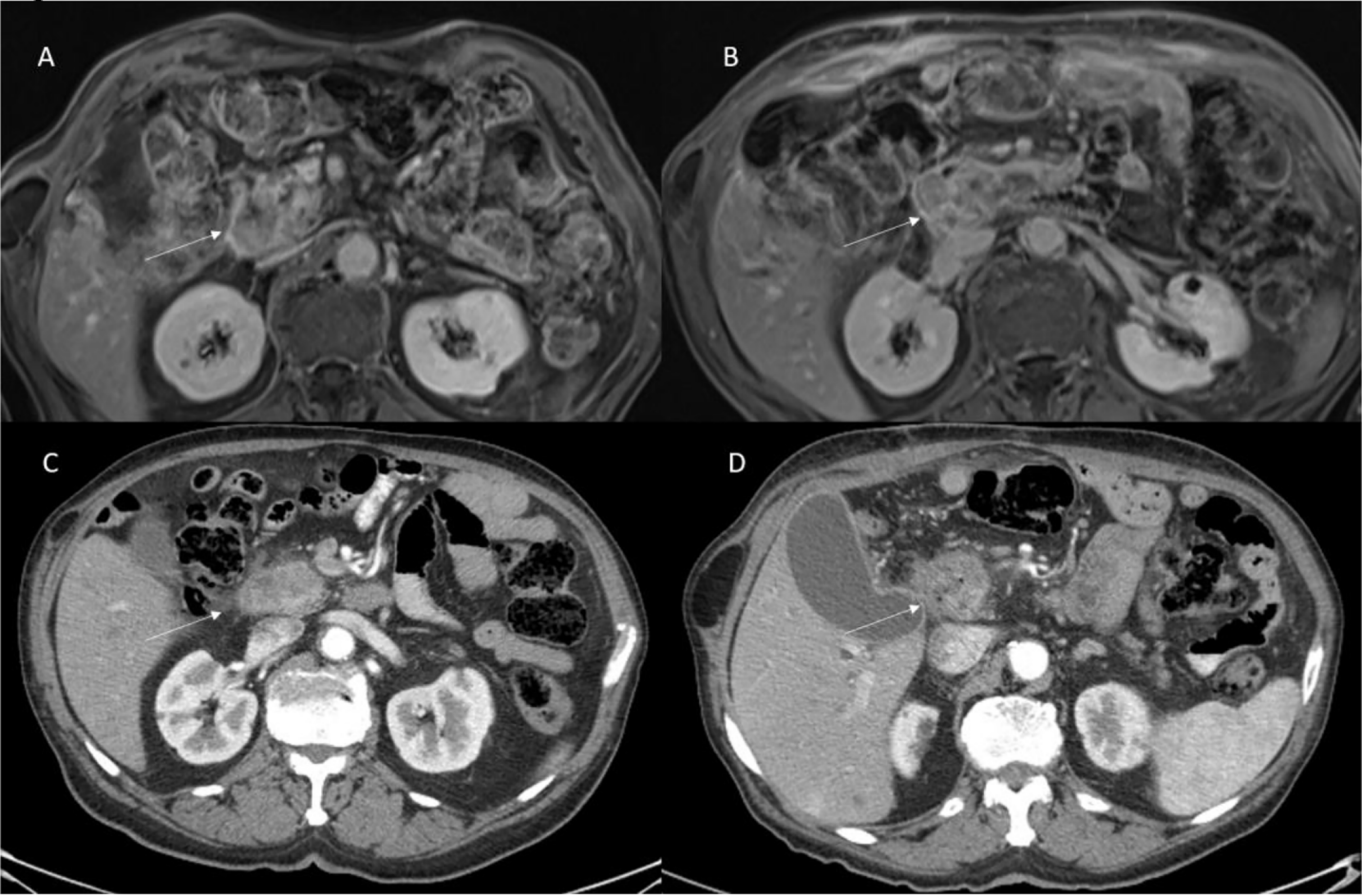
Patient 3 with head pancreatic cancer. Morphological MRI and CT assessment post-ECT treatment. In (A) (VIBE T1-W post-contrast sequence during portal phase in axial plane) and (C) (CT scan during pancreatic phase of contrast study) the arrow shows lesion. In (B) (VIBE T1-W post-contrast sequence during portal phase in axial plane) and (D) (CT scan during pancreatic phase of contrast study) the arrow shows ablated area. Qualitative assessment shows no significant differences in SI in pre- and post-treatment sequences and no significant differences in density in pre- and post-CT images.
